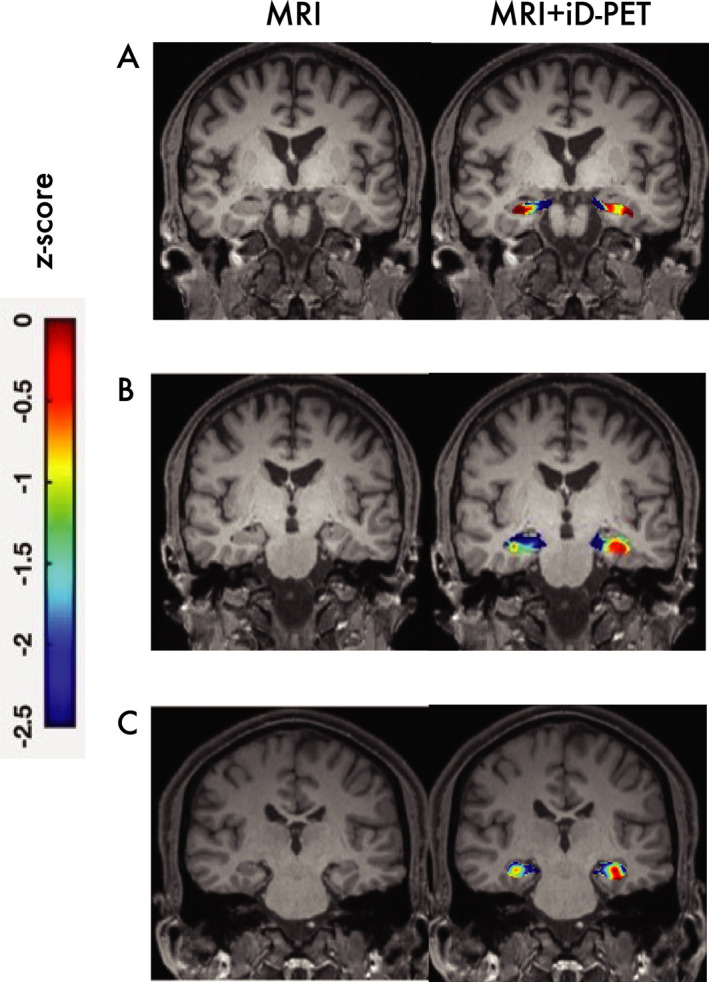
T1‐weighted MRI (left side images) and co‐registered dynamic FDG‐PET maps of hypometabolism by z score (right images) from epilepsy patients whose standard, static FDG‐PET images were normal. (A) This patient underwent a previous right anterior frontal lobotomy with no improvement in seizure frequency. iD‐PET demonstrated relative right hippocampal hypometabolism; subsequent intracranial monitoring confirmed seizure onset in the right hippocampus. (B) This patient had evidence of bilateral mesial temporal lobe epilepsy. iD‐PET disclosed worse right hippocampal hypometabolism; this patient declined further surgical intervention. (C) Patient with normal MRI and poorly localizing seizures with worse right hippocampal hypometabolism. This patient underwent thermal ablation of the right hippocampus and has long‐term seizure remission.^9^
